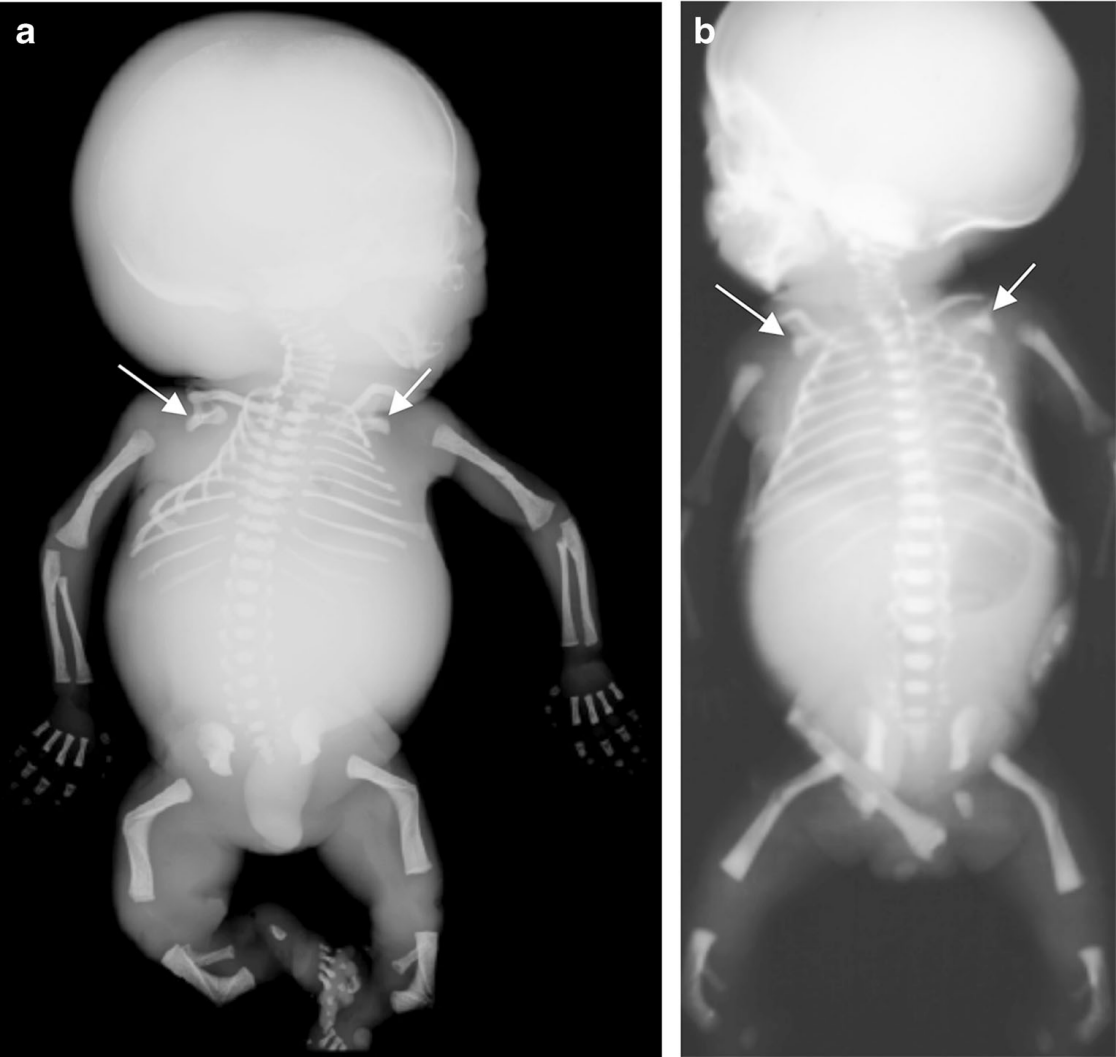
Radiographic features of lethal campomelic dysplasia in female and male fetuses*.*a,b Anteroposterior radiographs in female (a) and male (b) fetuses show 11 pairs of ribs, hypoplastic scapulae (*arrows*), absent ossification of thoracic pedicles, characteristic bowing/angulation of tibiae and fibulae, and narrow iliac wings. [Image (a) reproduced with permission from Radcliffe Publishing [38], page 249, case 13, image 13c]
